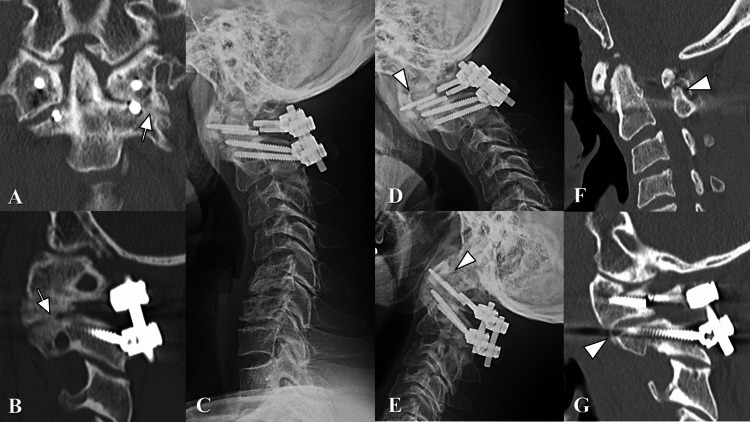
Postoperative images (continued). A and B (six months after surgery). Spontaneous fusion was detected at the left C1/2 facet joint (arrows) that originally had osteoarthritis in the coronal (A) and the sagittal (B) CT scan. C (12 months after surgery). Lateral plain radiography in standing position revealed kyphotic change at the subaxial cervical spine, which was already seen with spondylotic changes preoperatively, progressed slightly. D and E (12 months after surgery). Atlantoaxial interval did not change during neck flexion (D) and extension (E) (arrowheads). F and G (12 months after surgery). Although bony union was not observed between the posterior arch of C1 and the spinous process of C2 (F), further spontaneous fusion was detected at the right C1/2 facet joint by bridging of the anterior bony spur (G) in the sagittal CT scan.
